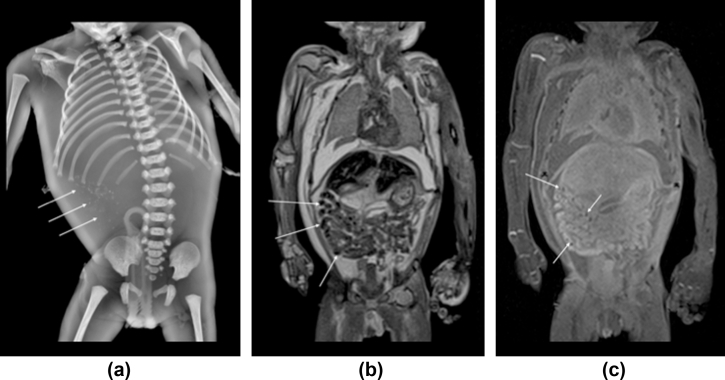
A 29-week-old stillborn fetus, found to have duodenal atresia and hypoplastic aortic arch at autopsy. The frontal PMSS (a) demonstrates multiple linear calcific densities in the right hemiabdomen. The subsequent post-mortem MRI images, presented in coronal section on the (b) T2-and (c) T1-weighted sequences demonstrate low signal intraluminal material (arrows), consistent with calcified meconium.
